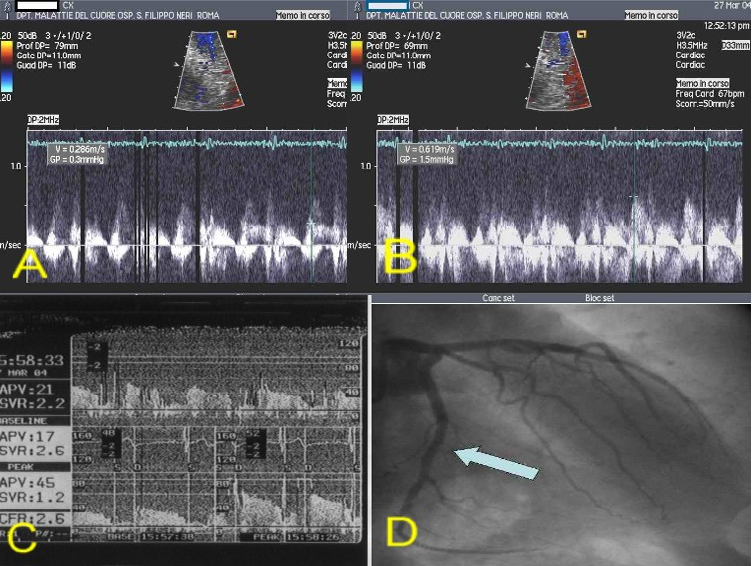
Patients 3. A: TTDE before adenosine. B: TTDE after adenosine. C: DW recording. D: coronary angiography with site of DW recording (arrow). DW: Doppler-wire. TTDE: transthoracic Doppler echocardiography.
